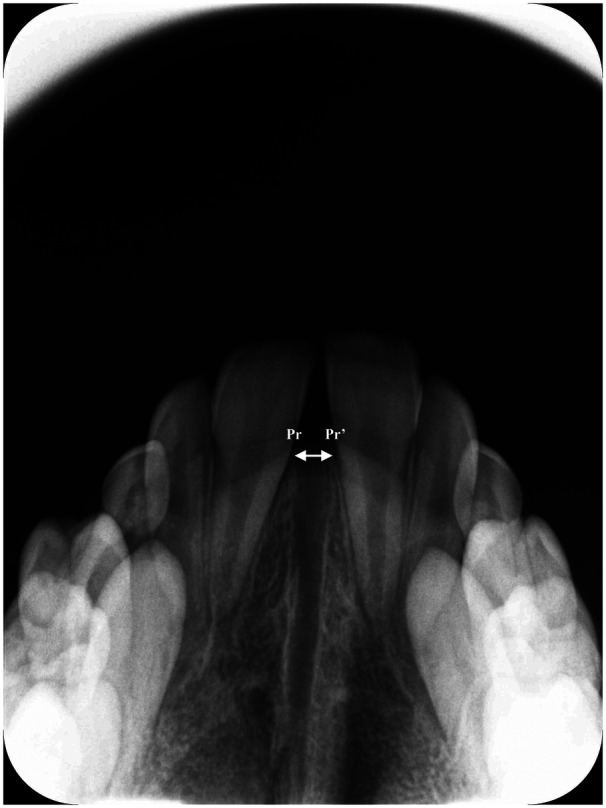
The amount of midpalatal suture separation was quantified by calculating the distance between the prosthion points (Pr–Pr′) on maxillary occlusal radiographs.
